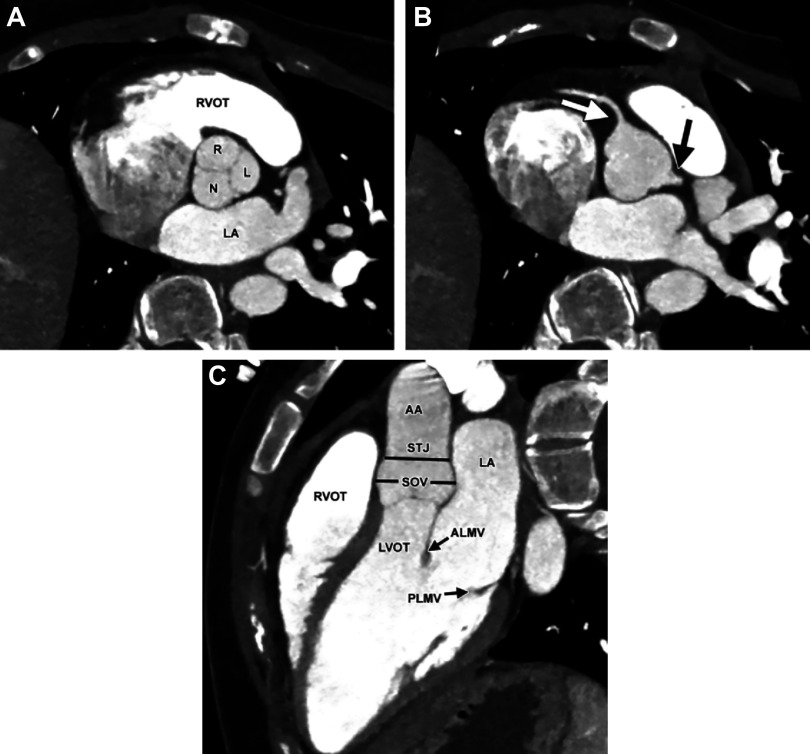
Normal aortic valve (AV) apparatus (AVA) anatomy by cardiac computed tomography (CT) imaging. *A*: double oblique CT image at the AV level with right (R), left (L), and noncoronary (N) cusps. LA, left atrium; RVOT, right ventricular outflow tract. *B*: double oblique CT image at the coronary ostia level. The white and black arrows point to the coronary arteries at their ostia. *C*: long-axis cardiac CT image showing a 3-chamber view. AA, ascending aorta; ALMV, anterior leaflet of the mitral valve; LVOT, left ventricular outflow tract; PLMV, posterior leaflet of the mitral valve; SOV, sinuses of Valsalva; STJ, sino-tubular junction. Adapted from Ref. 226, with permission from *RadioGraphics*.
